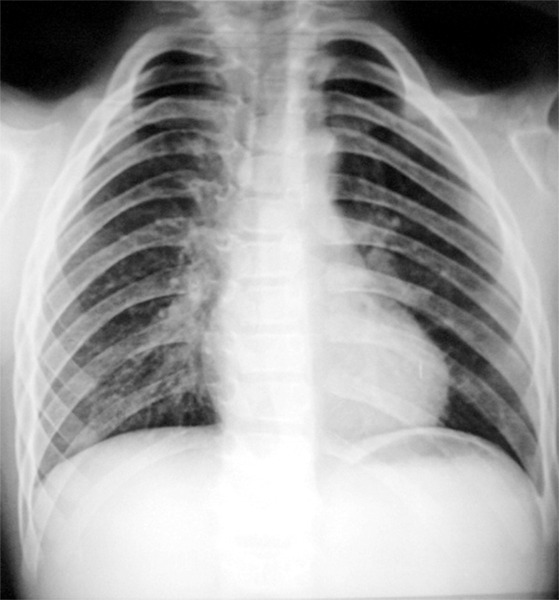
X-ray PA view chest of the 9-year-old male with cleidocranial dysplasia, showing laterally deficient clavicles
